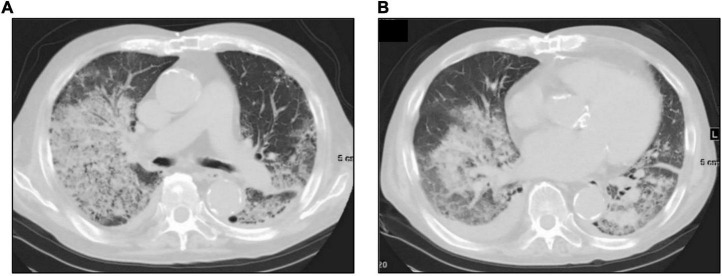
High-resolution computed tomography of the chest on admission. Chest computed tomography on admission shows diffuse “ground-glass” opacities (A), consolidation, cardiac enlargement, and bilateral pleural effusion (B).
